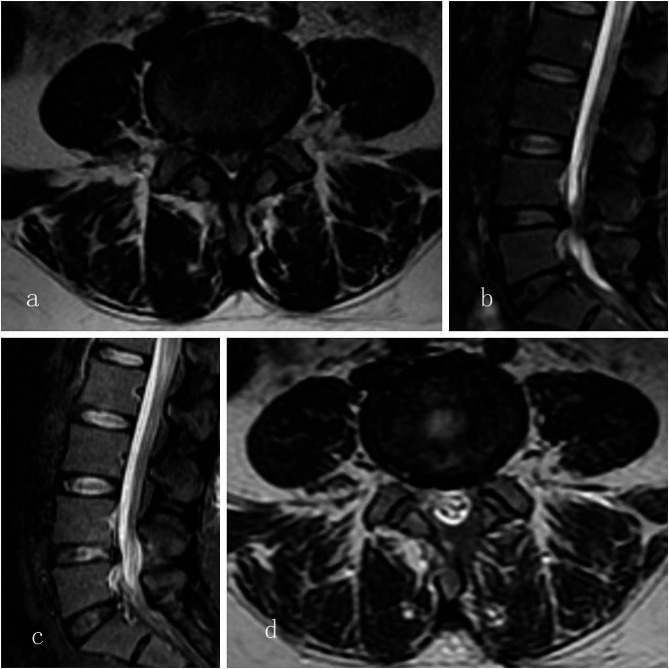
a and b show preoperative horizontal and sagittal MRI images, respectively, while c and d represent postoperative horizontal and sagittal MRI images, respectively, indicating a significant increase in the cross-sectional area of the spinal canal
